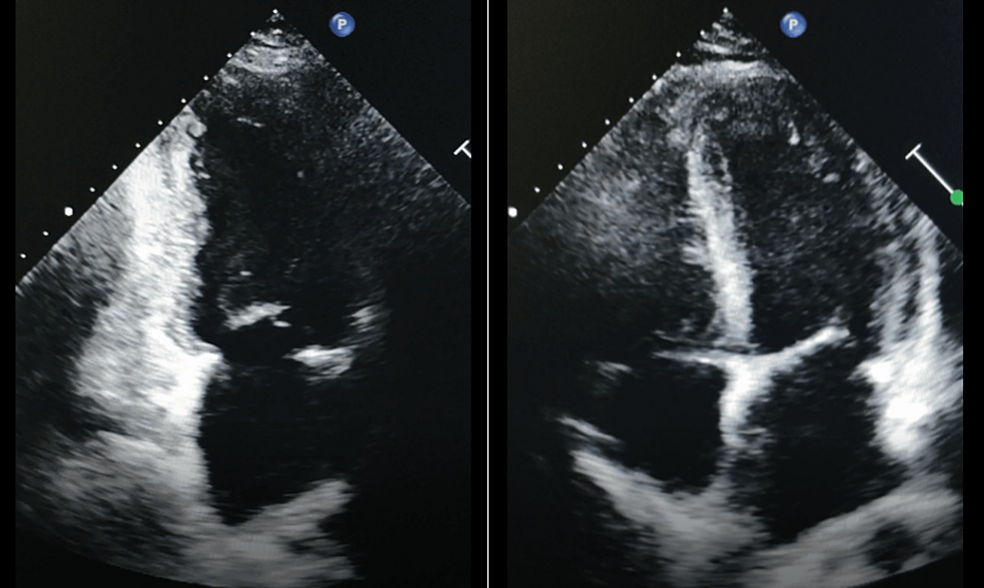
TTE showing the continuity of the myocardial wall without apparent structural abnormality. TTE: transthoracic echocardiography.
